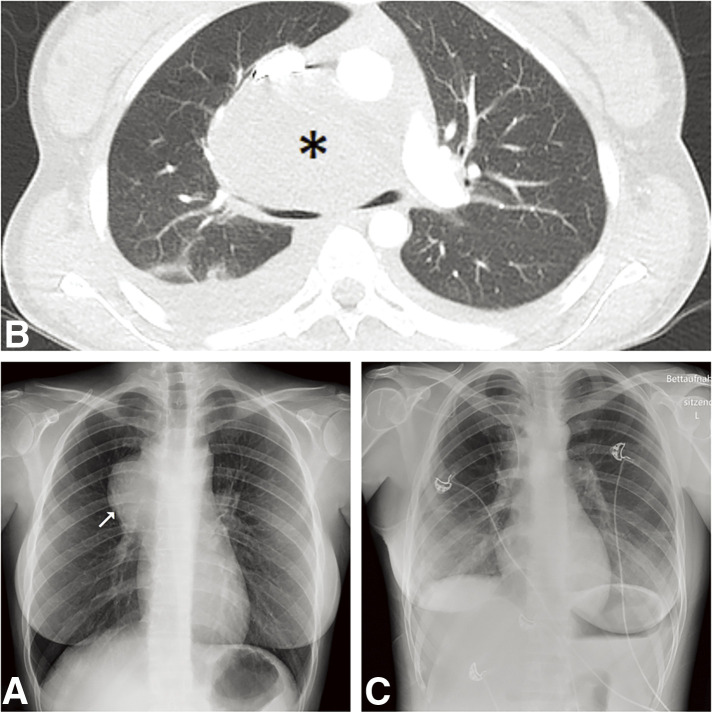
(A) Chest X-ray showing on the right side the bronchogenic cyst as a round mass in the anterior upper mediastinum (arrow). Differential diagnoses include lymphoma, thymoma, germ cell tumor, or an intrathoracic deeply located goiter. (B) CT scan showing a mediastinal mass compressing the right upper lobe artery, superior vena cava, and the main bronchi (asterisk) (C) Chest X-ray after the removal of the mediastinal bronchogenic cyst.
